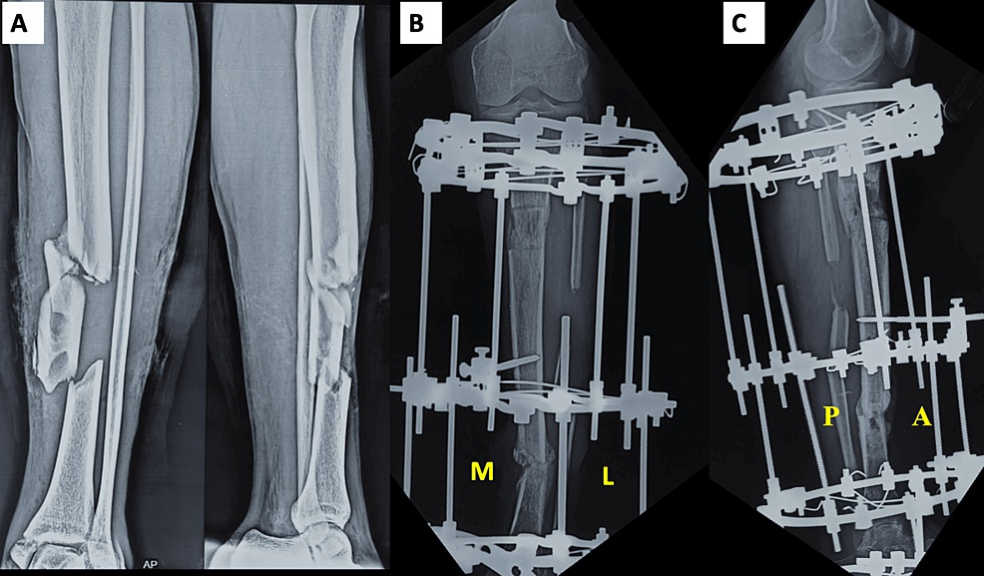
Application of the m-RUST score in the tibia diaphysis fracture treated by the Ilizarov frame fixator. A) Pre-operative X-ray of a segmental tibia fracture that was treated with excision of the infected segmental fragment and Ilizarov fixator application with bone transport. B) AP view of the leg with the medial cortex having no callus (score = 1), lateral cortex having bridging cortex (score = 3). C) Lateral view of the leg with the anterior cortex remodelled with no visible callus (score = 4) and posterior cortex showing bridging callus (score = 3). The total m-RUST score of 11. AP = Anterio posterior; X-ray = Radiograph; m-RUST = Modified Radiological Union Score of Tibia; AP = Anterioposterior; M = Medial; L = Lateral; A = Anterior; P = Posterior
